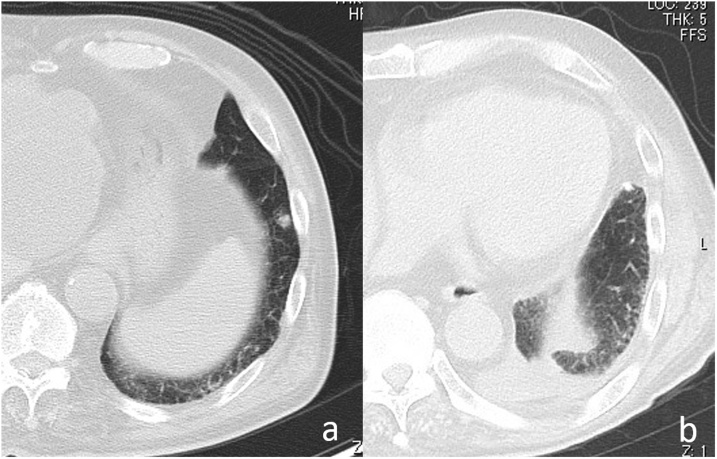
MTX-associated lymphoproliferative disorder in the left lower lobe. [a] Chest computed tomography (CT) at 2 weeks after the first surgery showing a new 1-cm-sized nodule in the left lower lobe. [b] Chest CT at 1 month after the second surgery showing that the nodules in the left lower lobe have disappeared.
